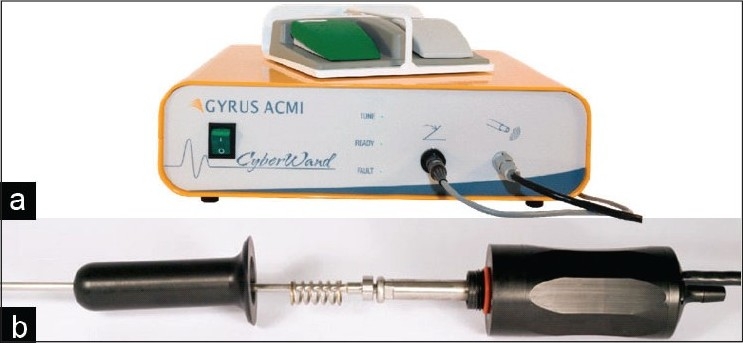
CyberWand^®^ dual probe ultrasonic lithotripter with dual energy foot pedal sitting atop generator (a.) and disassembled handpiece (b.). Note: parts on handpiece from left to right include spring, floating probe, free ring, and fixed probe
